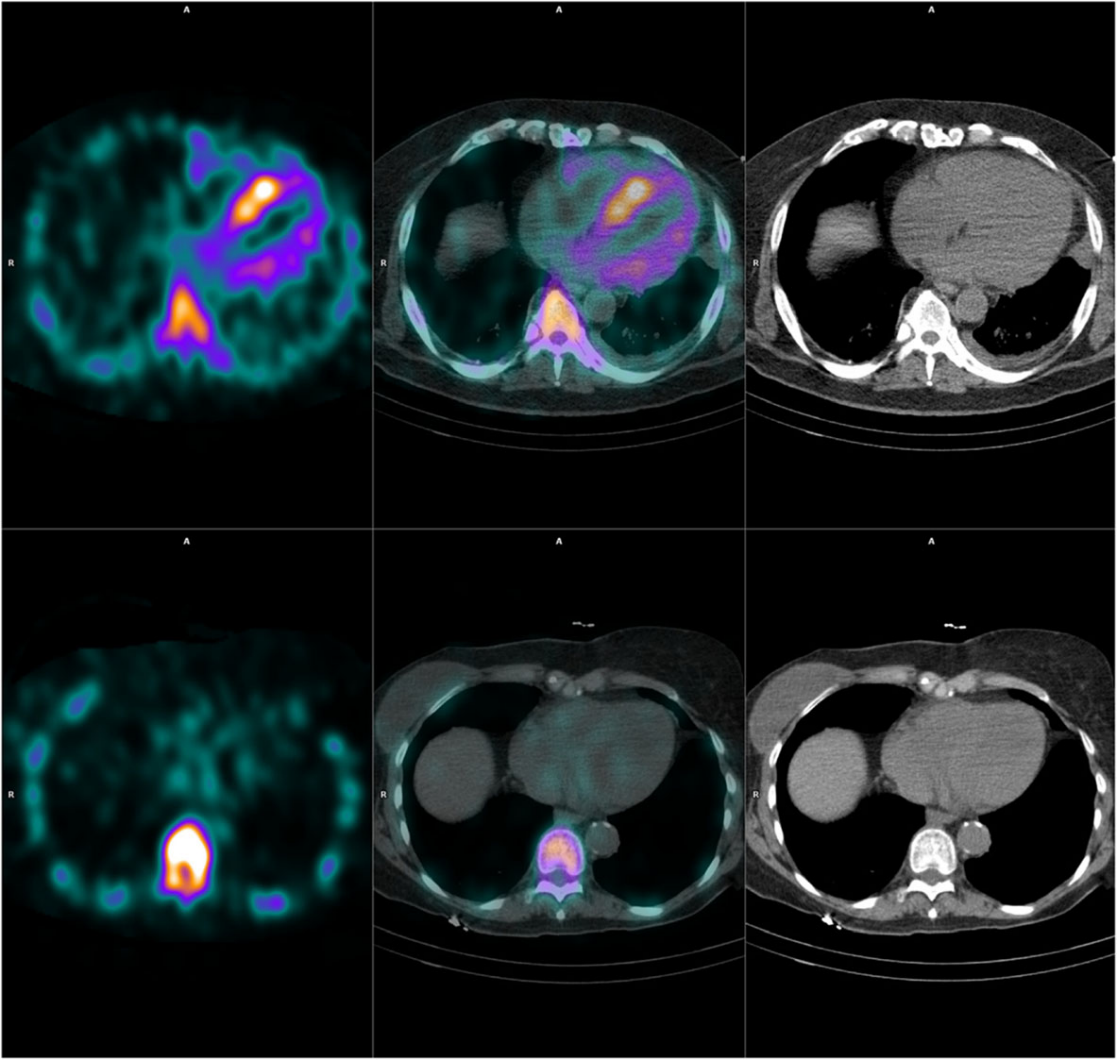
Examples of xSPECT/CT transaxial images through the heart at midventricular level. The upper row is from a patient with ATTR cardiac amyloid, planar imaging in this individual showed Perugini grade 2. The lower row is from a patient with no known heart disease (history of previous right breast cancer)
